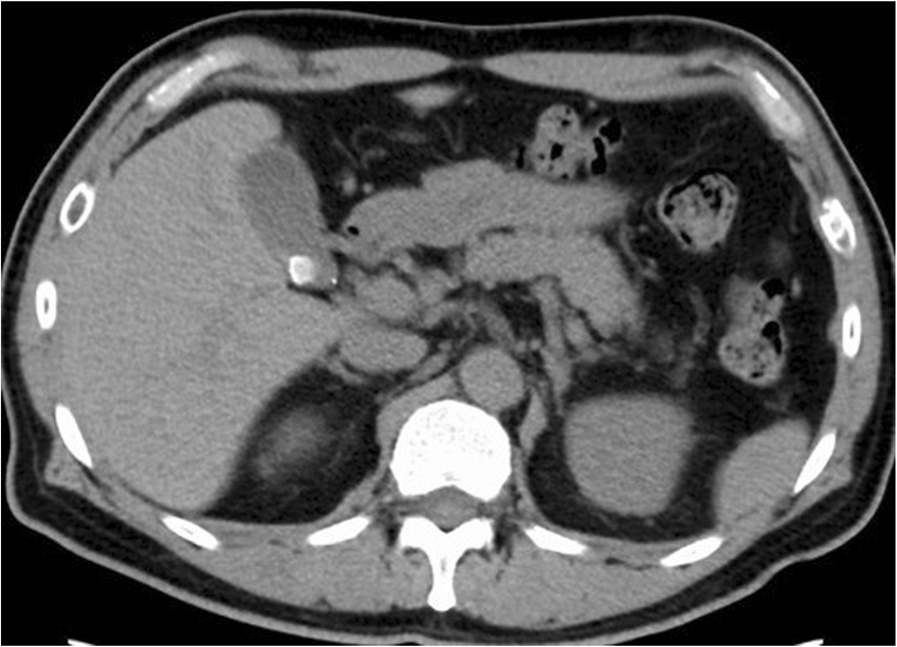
Computed tomography showing calculous cholecystitis (axial sections)
